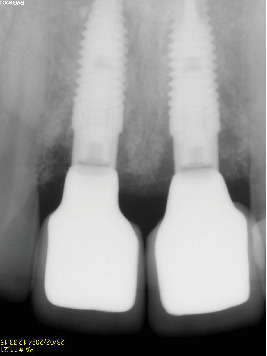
The 3-year follow-up radiograph shows no bone loss around the implants.
